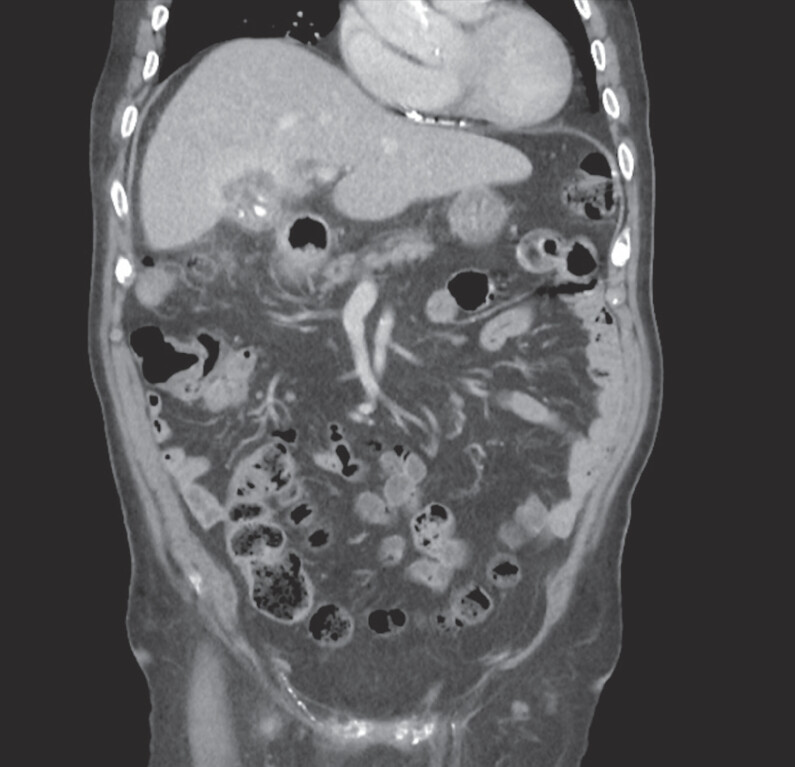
An abdominal computed tomography revealed acute cholecystitis
